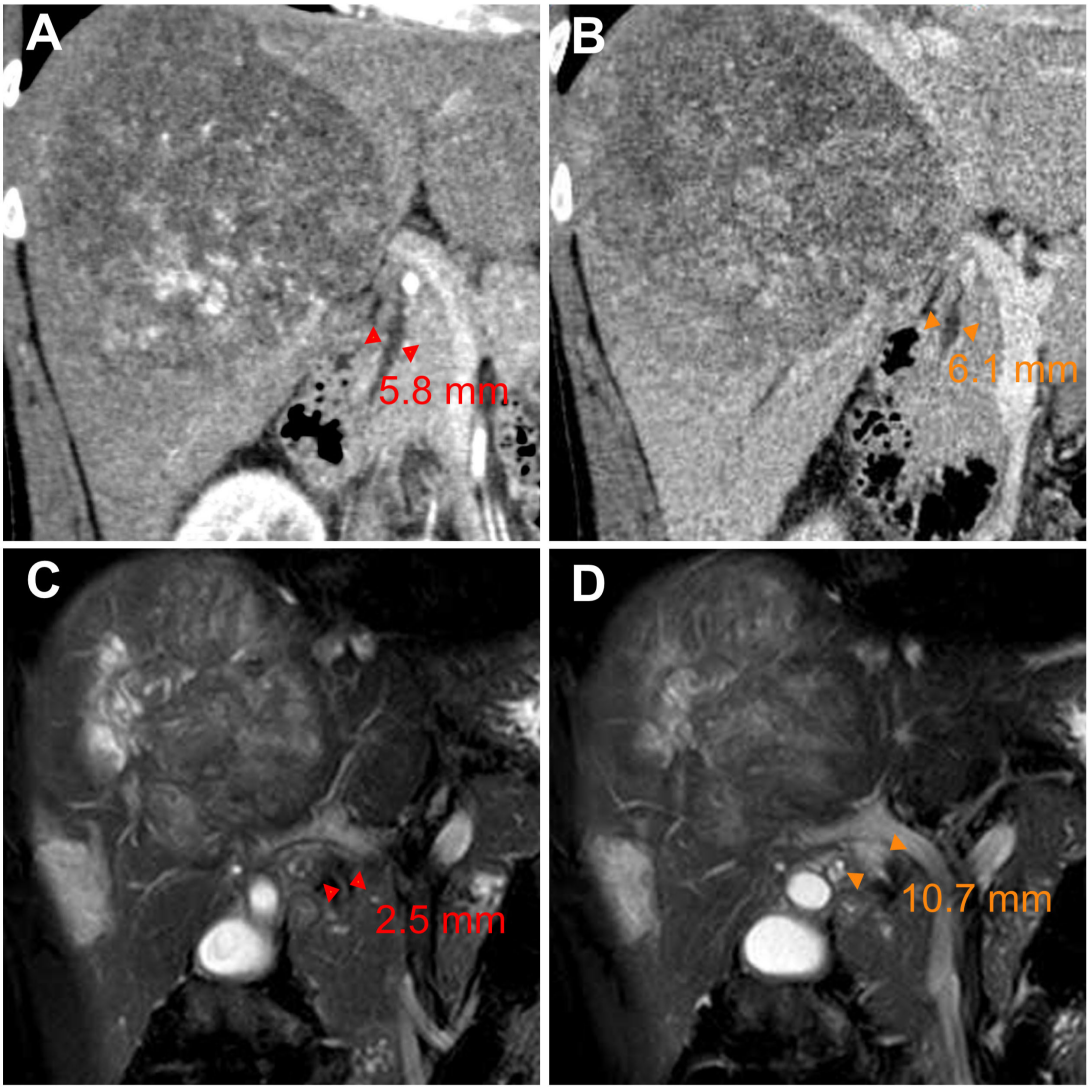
Radiologic scans of the common bile duct before and after conversion therapy. (A, B) Coronal multiplanar reconstruction of contrast-enhanced computed tomography images displaying the pretreatment (baseline) diameter of the middle-lower (A) and upper (B) portions of the common bile duct. (C, D) Magnetic resonance cholangiopancreatography images revealing (C) stricture of the middle-lower portion of the common bile duct and (D) dilatation of the upper biliary region on day 155 from baseline. ▸◂, duct diameter as measured by imaging.
